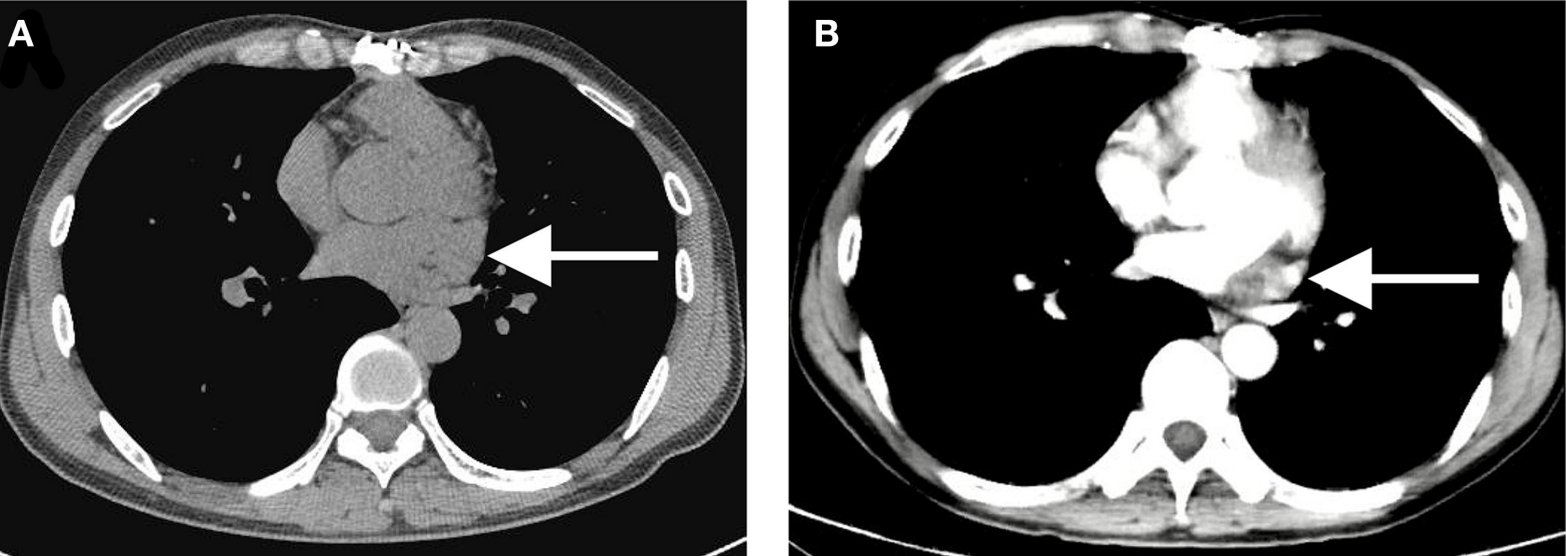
A 2- (A) to 4-year (B) post-operative computed tomography showing no evidence of remnant lymphangiohemangioma progression with the same size of 10 × 15 × 30 mm (arrow).
